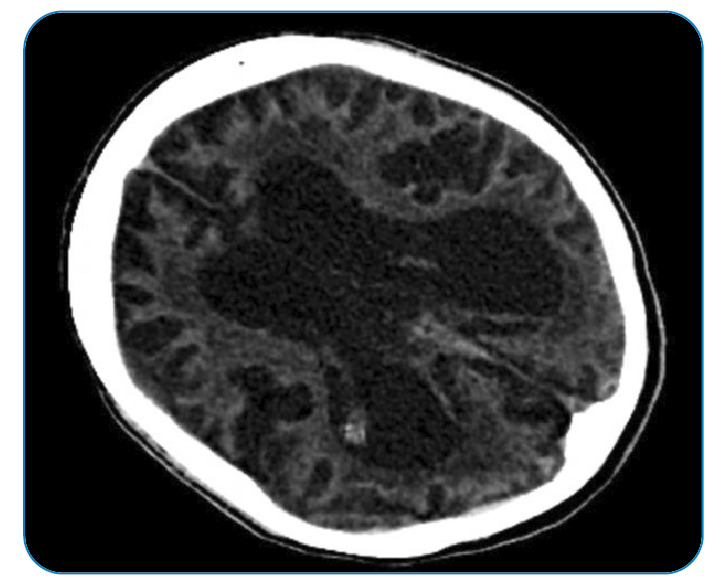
Diffuse cortical atrophy on the brain CT
